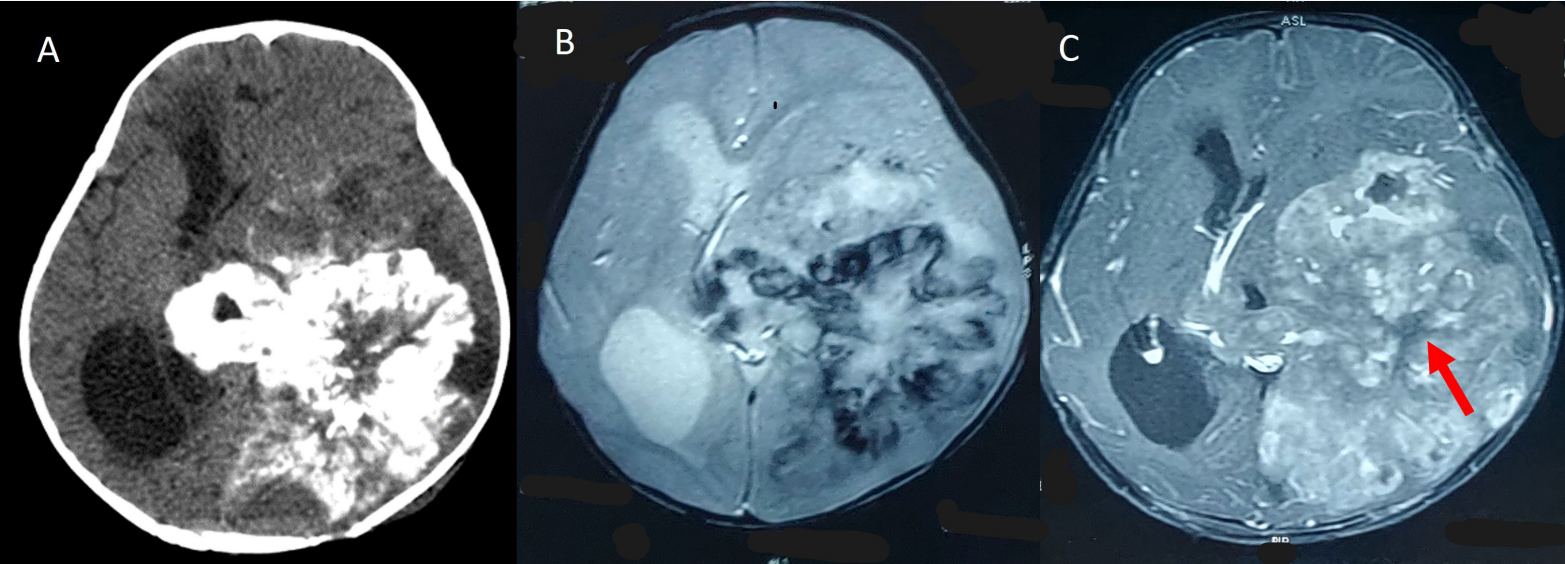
Periwinkle sign. A. Axial noncontrast-enhanced CT image showing a large heterogeneous mass in the left temporoparietal lobe in the periventricular location, with dense centripetal calcification giving rise to a flower like shape; B. axial SWI shows a similarly shaped area of blooming; C. contrast-enhanced T1W MR image shows a central non-enhancing area of necrosis (red arrow)
